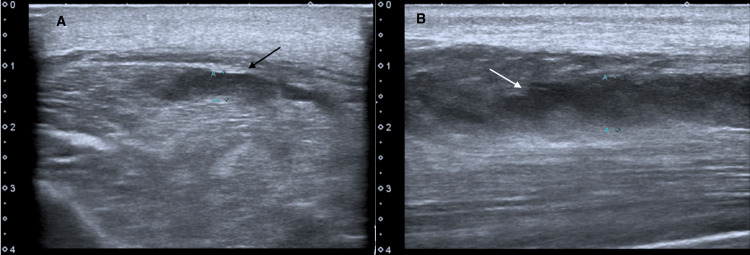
Transverse (Panel A) and longitudinal views (Panel B) of the right leg ultrasound showing muscle tissue heterogeneity and areas of liquid collection (arrows)
